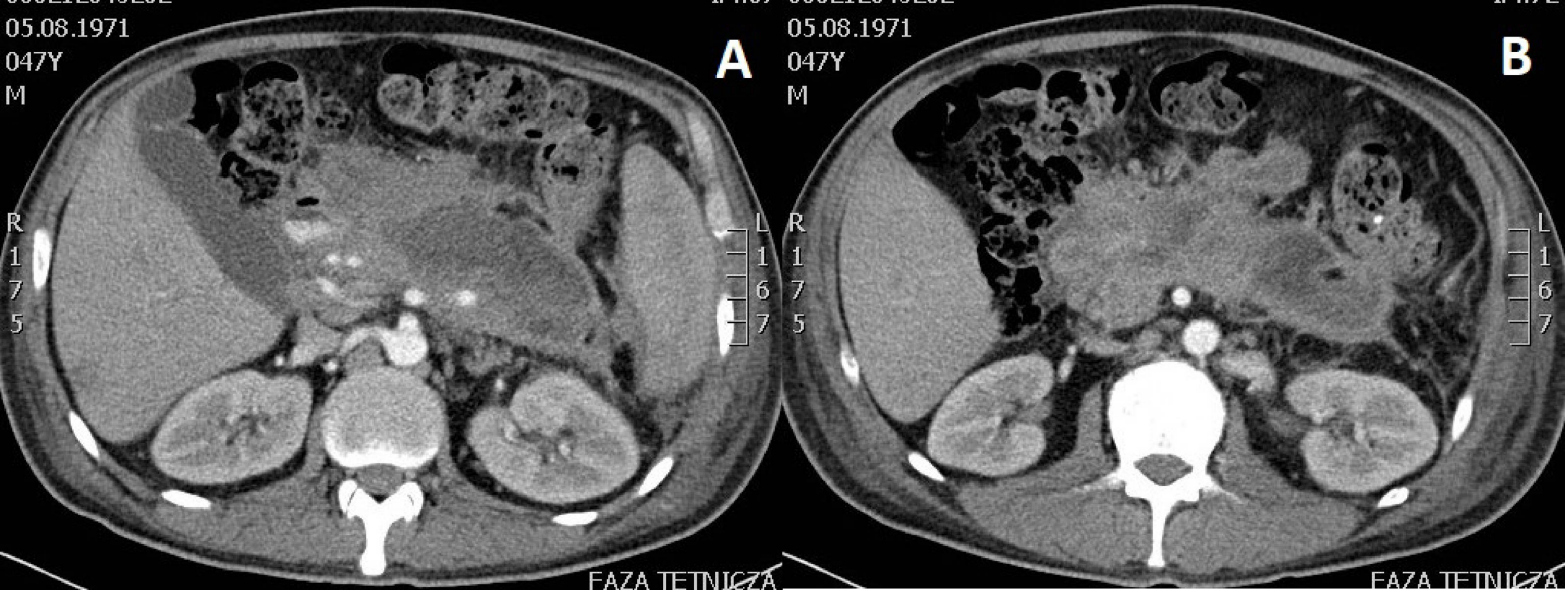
(A, B). Abdominal contrast-enhanced computed tomography scan performed in a patient with infected walled-off pancreatic necrosis six weeks after acute necrotic pancreatitis of alcoholic etiology. Gas bubbles seen within the lumen provide an indirect proof of infected fluid collection after excluding spontaneous fistulization of pancreatic necrosis to the gastrointestinal tract.
